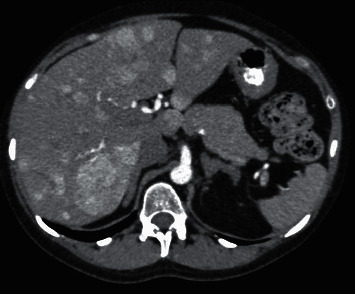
CT of the abdomen showing multiple irregular lesions in the liver, with the largest measuring 6.0 × 6.0 cm (white arrow).
